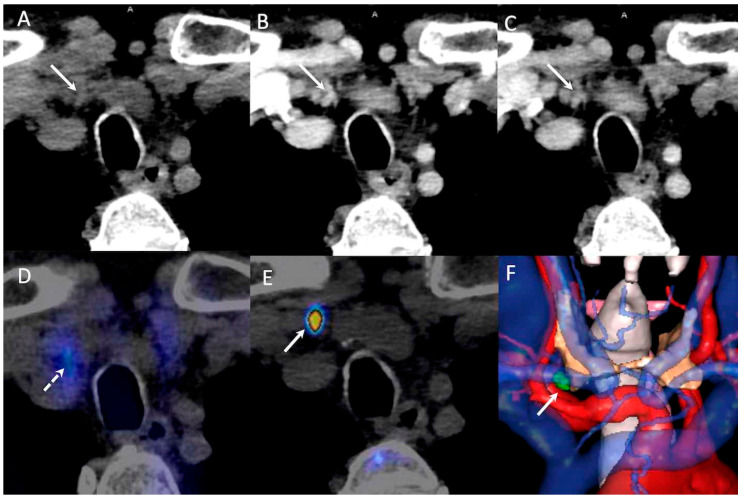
79-year-old man with primary hyperparathyroidism (pHPT) and inconclusive neck ultrasonography (US) and parathyroid scintigraphy. Positron emission tomography/four-dimensional computed tomography (PET/4D-CT) identified one right retro-clavicular nodule with typical contrast-media enhancement profile and high ^18^F-FCH uptake (arrow). Pathology confirmed a lower parathyroid adenoma (0.2 g, 10 mm) descending into the superior mediastinum in the right thyrothymic ligament. (A–C): four-dimensional computed tomography (4D-CT), axial slice: no-contrast-media computed tomography (CT), 45 HU; 45 sec post-injection, 90 HU; 70 sec post-injection, 63 HU. (D): parathyroid scintigraphy, axial slice. (E): ^18^F-Fluorocholine positron emission tomography (^18^F-FCH PET), axial slice. (F): 3D-virtual neck exploration, anterior view; green: right lower parathyroid, orange: thyroid.
